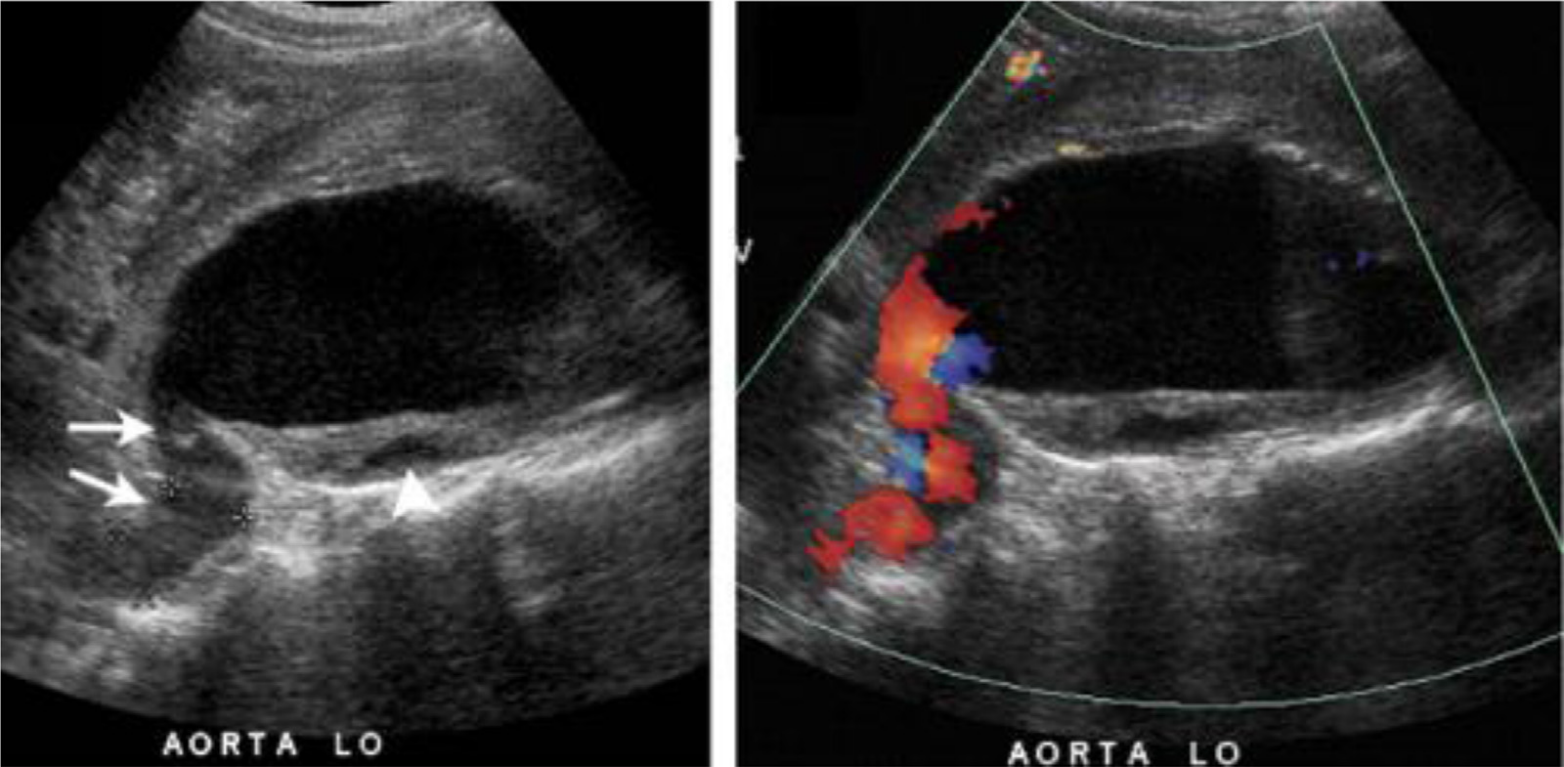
Bedside ultrasound revealed 8 cm AAA.
